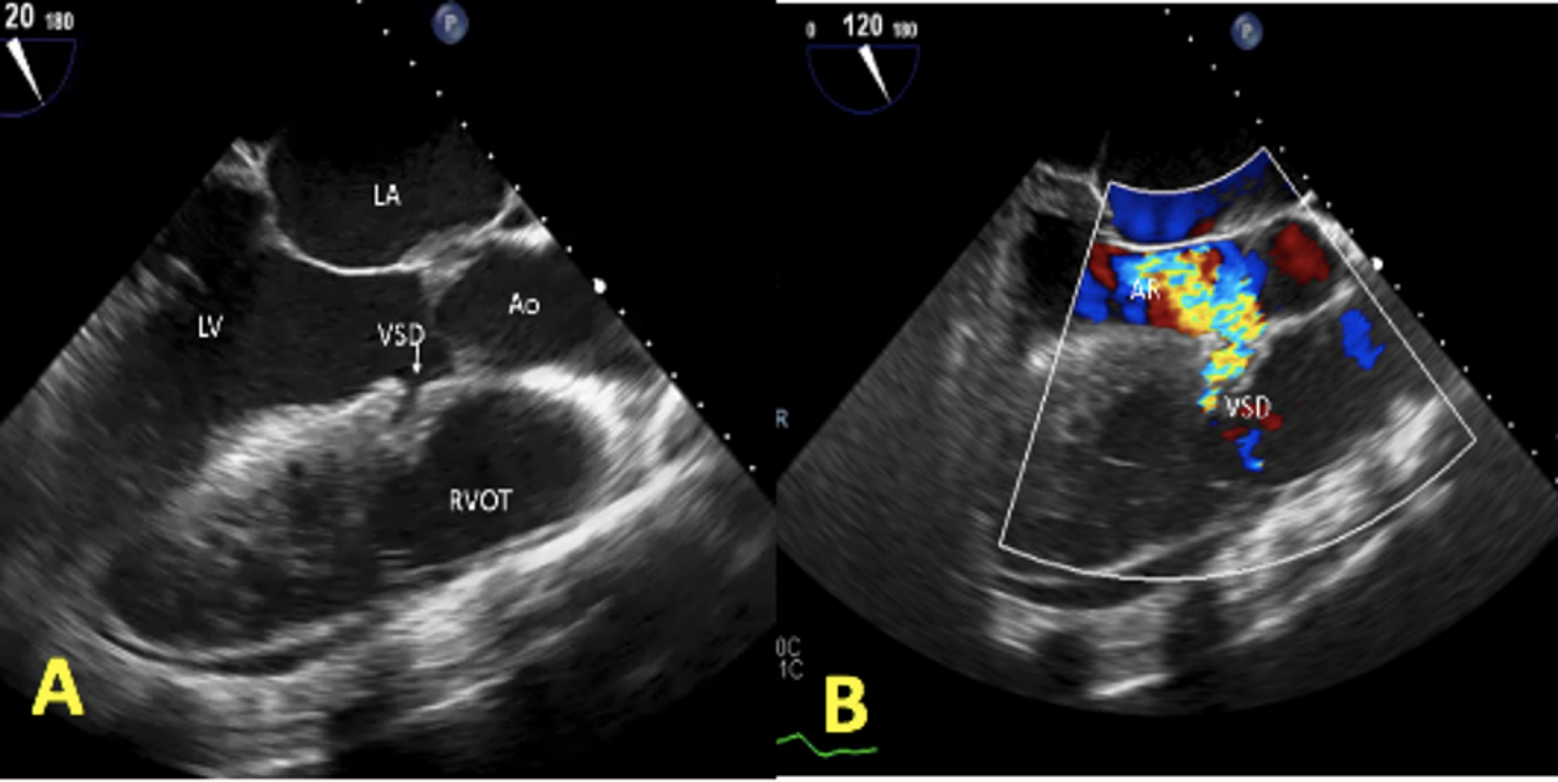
Two-dimensional transesophageal echocardiogram (TEE) images showing the small ventricular septal defect (VSD) in the upper part of the septum with a left to right shunt (A), and severe aortic insufficiency (B)
